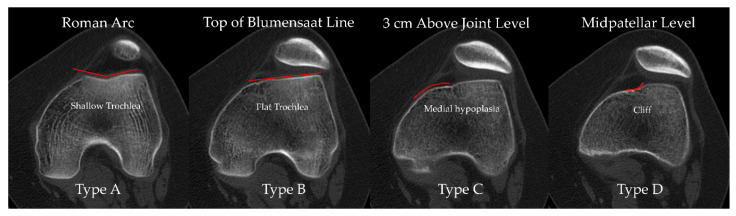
Axial CT images from the same patient demonstrate how Dejour trochlear dysplasia grading varies according to the axial level. From left to right: at the Roman arc level the trochlea appears shallow and would be classified as Type A; at the level of the top of the Blumensaat line it appears flat (Type B); at 3 cm above the joint line there is medial trochlear hypoplasia consistent with Type C; and at the midpatellar level a “cliff” pattern suggests Type D. Red lines outline the trochlear articular surface and highlight the level-dependent variation in morphology.
